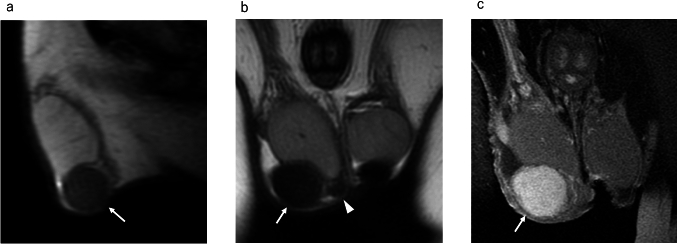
Adenomatoid tumor in a 43-year-old male. a Sagittal T2-weighted MR image shows a hypointense mass (white arrow) measuring 2.5 cm. The testis is compressed by the mass without invading the testis parenchyma, indicating that it is an extratesticular mass. b Coronal T2-weighted MR image shows a hypointense mass (white arrow) which is suspected to be continuous with the tail of the epididymis (white arrowhead). c Coronal contrast-enhanced fat-suppressed T1-weighted MR image shows homogeneous enhancement of the mass (white arrow)
